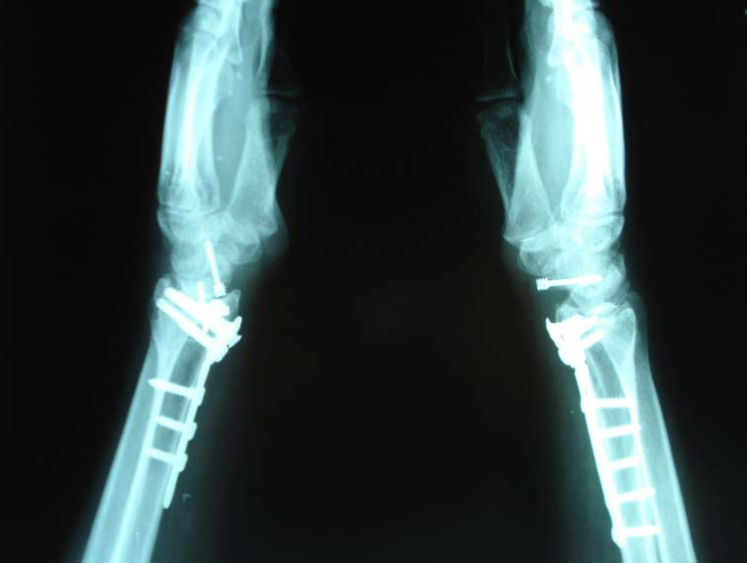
Postoperative lateral roentgenogram of the right and left distal radius and scaphoid at 3 months.
